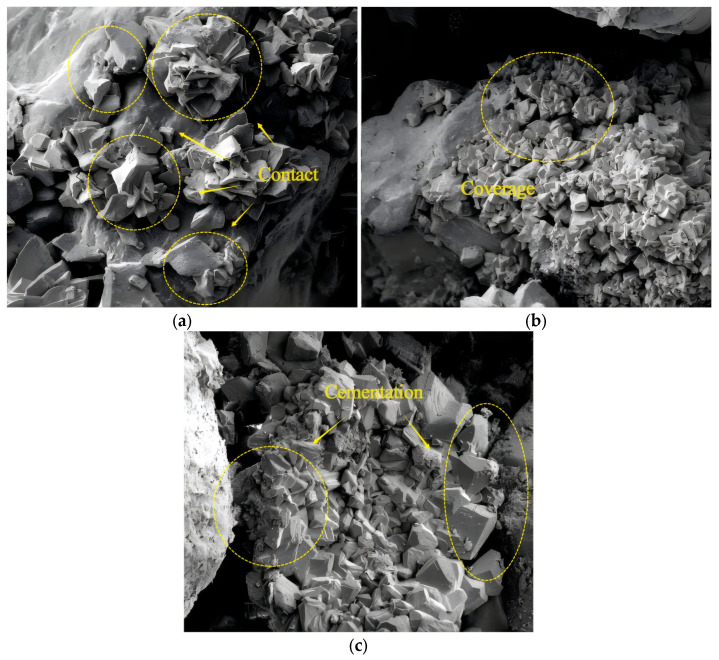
SEM image: (a) *CT* = 2; (b) *CT* = 4; and (c) *CT* = 6.
